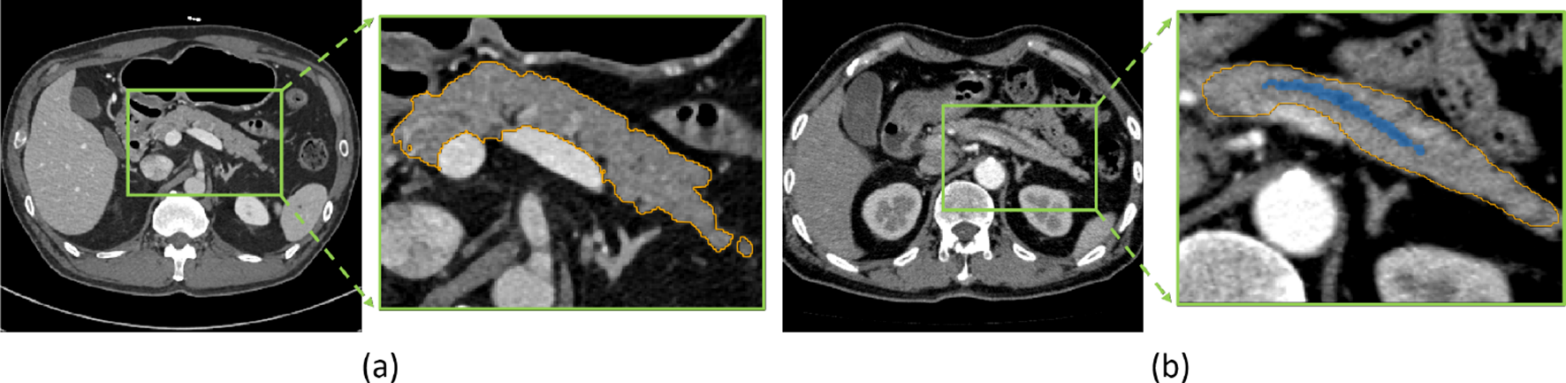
Visual examples of axial CT slices of a normal pancreas and b pancreas with dilated pancreatic duct. The pancreas region is surrounded by the orange contour, and the pancreatic duct is indicated by blue
